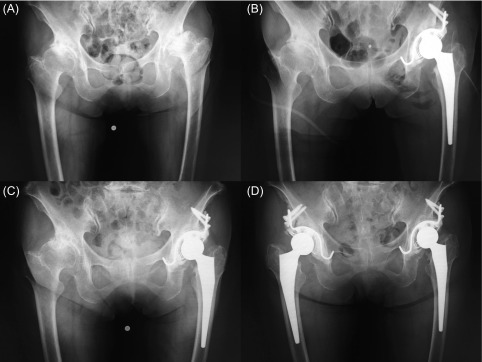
(A) Osteoarthritis of the bilateral hip, (B) immediate post-operative x-ray of the left hip, (C) 12 months after the operation. Right hip required THA, (D) there is no sign of implant loosening at 12 month after right THA, 24 months after left THA.
